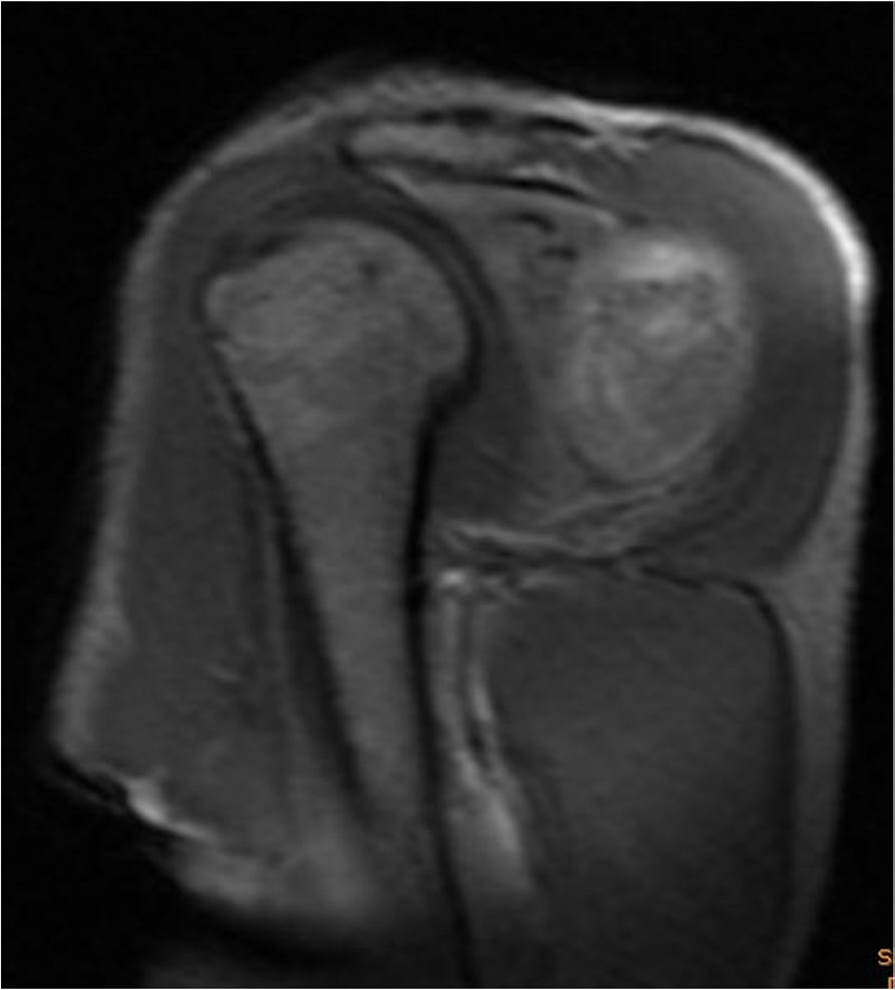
MRI of the right shoulder shows that tumor mass was 13.2 × 4.1 × 7.8cm in size, uneven long T2 and long T1 signal mass behind the right shoulder joint and deep in the deltoid muscle
